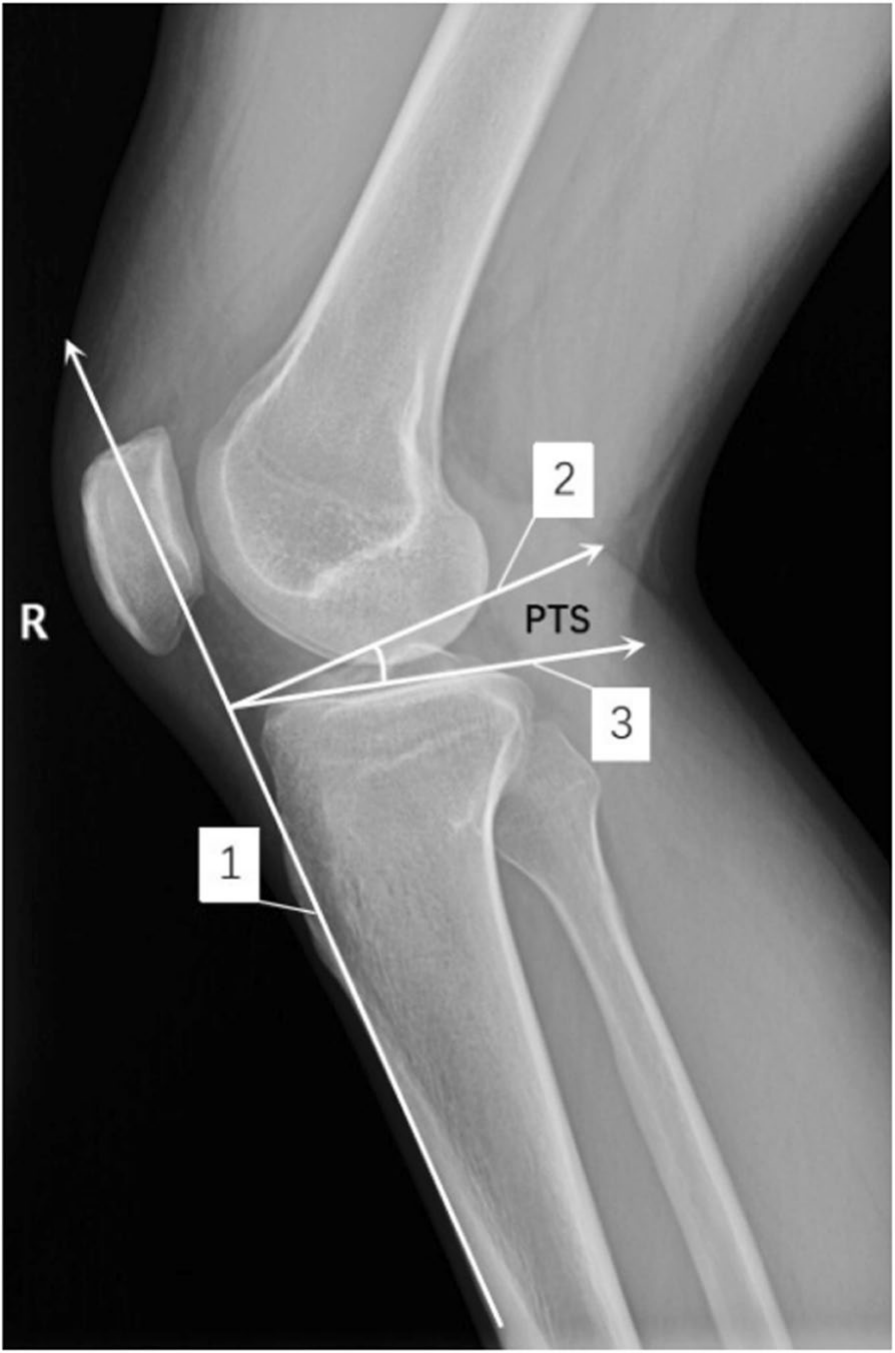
The measurement method of posterior tibial slope (PTS)^*^. ^*^X-ray lateral inspection: line 1 is the tangent line of the proximal tibia on the anterior cortex surface, line 2 is the perpendicular to line 1, and line 3 is the tangent line of the tibial plateau
